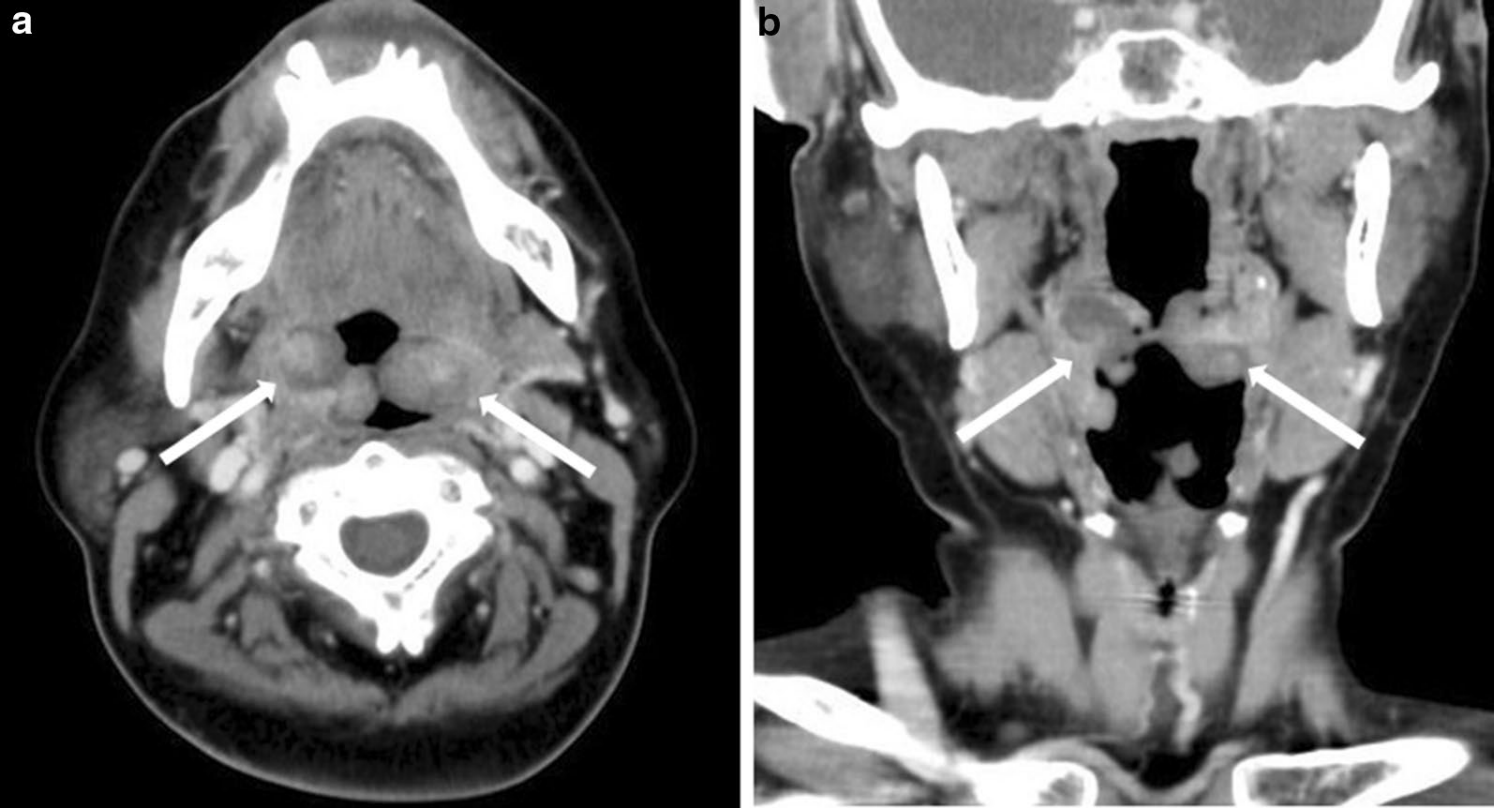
Neck CT findings: the CT images showed a tonsillar lesion with contrast enhancement, and tonsillar metastasis was suspected (a transverse plane, b coronal plane)
